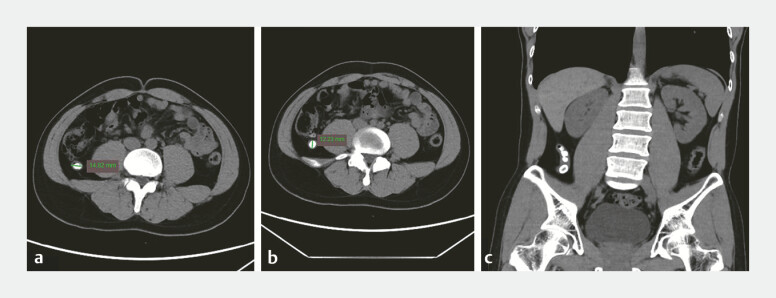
CT imagings prior to EDAT.
